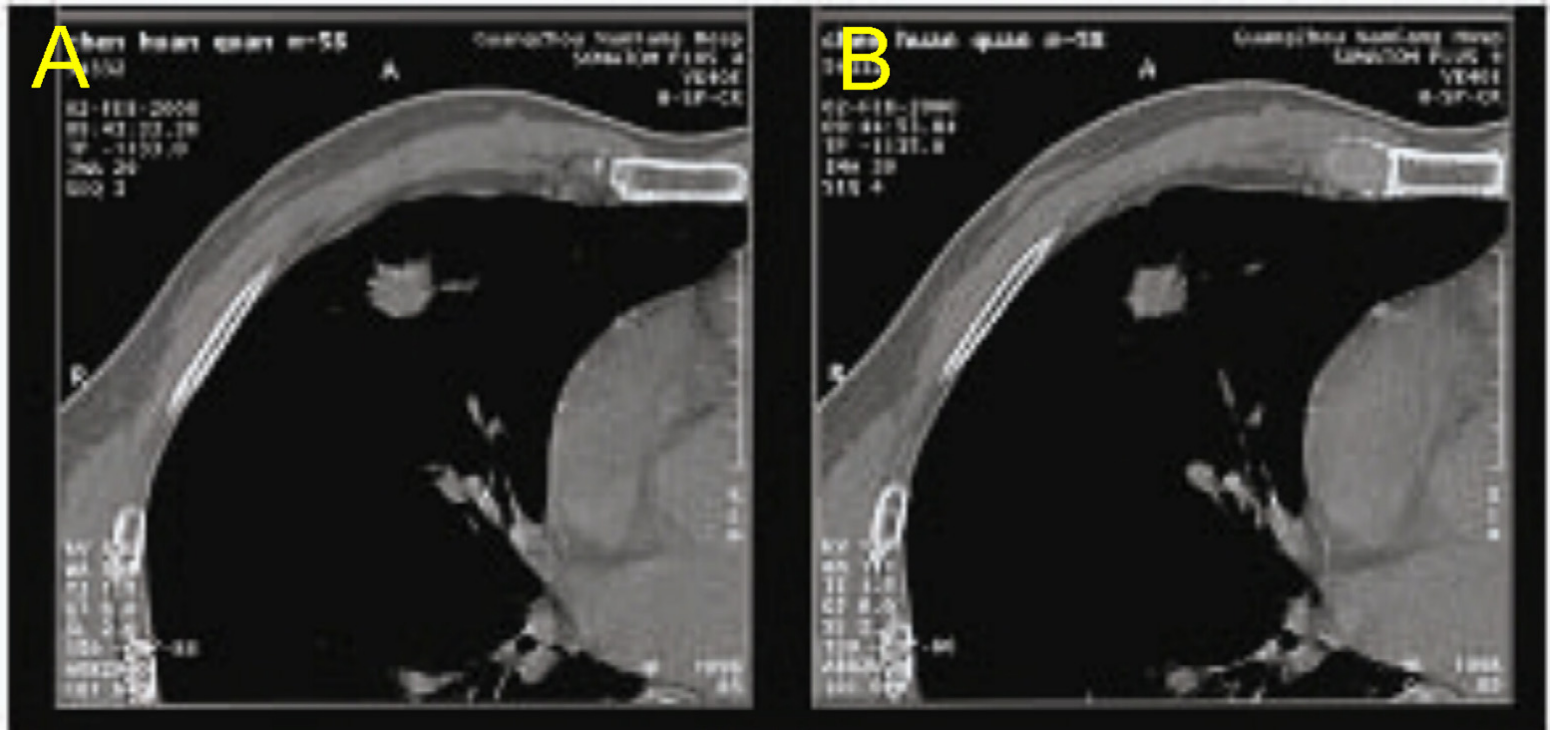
CT images taken before and after RFA treatments. The same patient PET images were shown as Figure 1. (A) was taken before RFA treatment, (B) was taken 2 week after RFA treatment.
